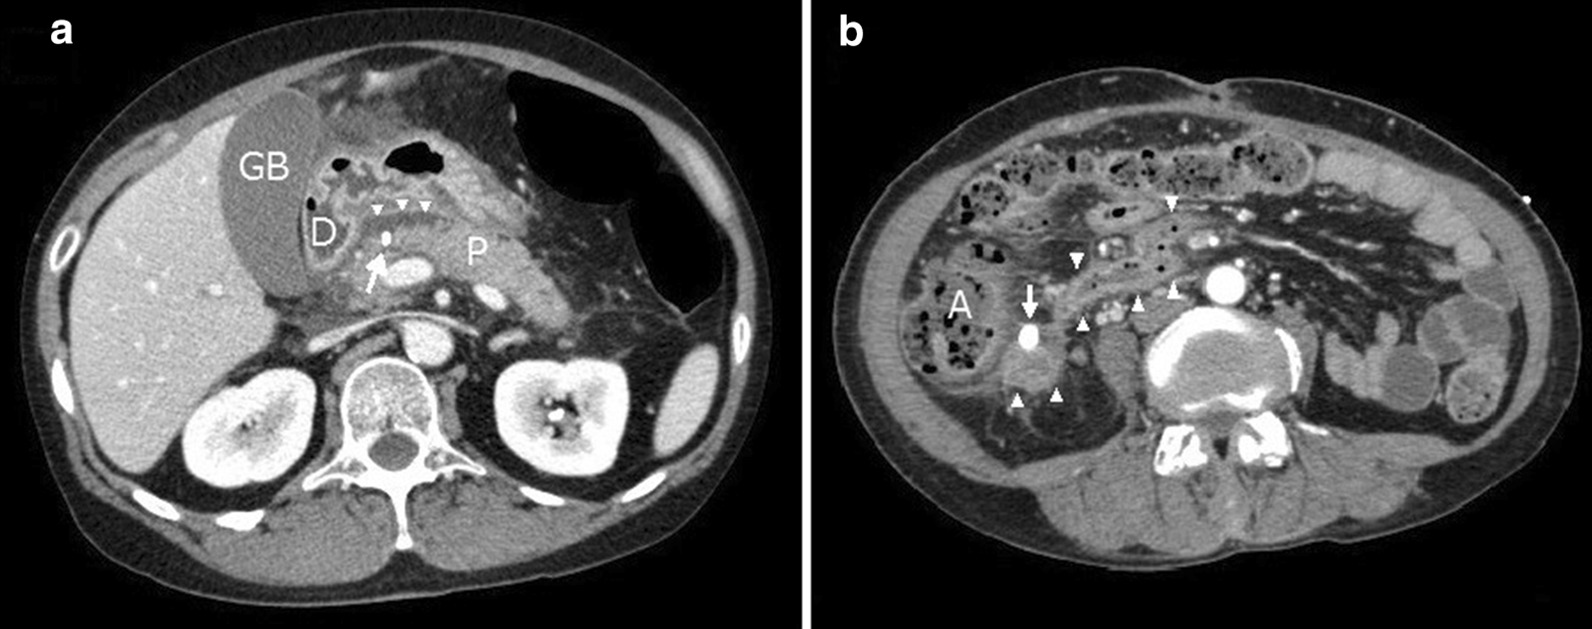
CT scans of the initial damage to the MPD and prolonged pancreatic leakage. a POD 1. The pancreatic duct stent tube is dislocated and protruding into the peritoneal cavity (arrow), and fluid collection over the pancreatic surface (arrowhead) is evident. b POD 80, before the third operation. Pancreatic fluid surrounded with inflamed tissue spread toward lower peritoneal space (arrowhead). A drainage tube (arrow) was placed near that cavity. *GB* gallbladder, *D* duodenum, *P* pancreas, *A* ascending colon
